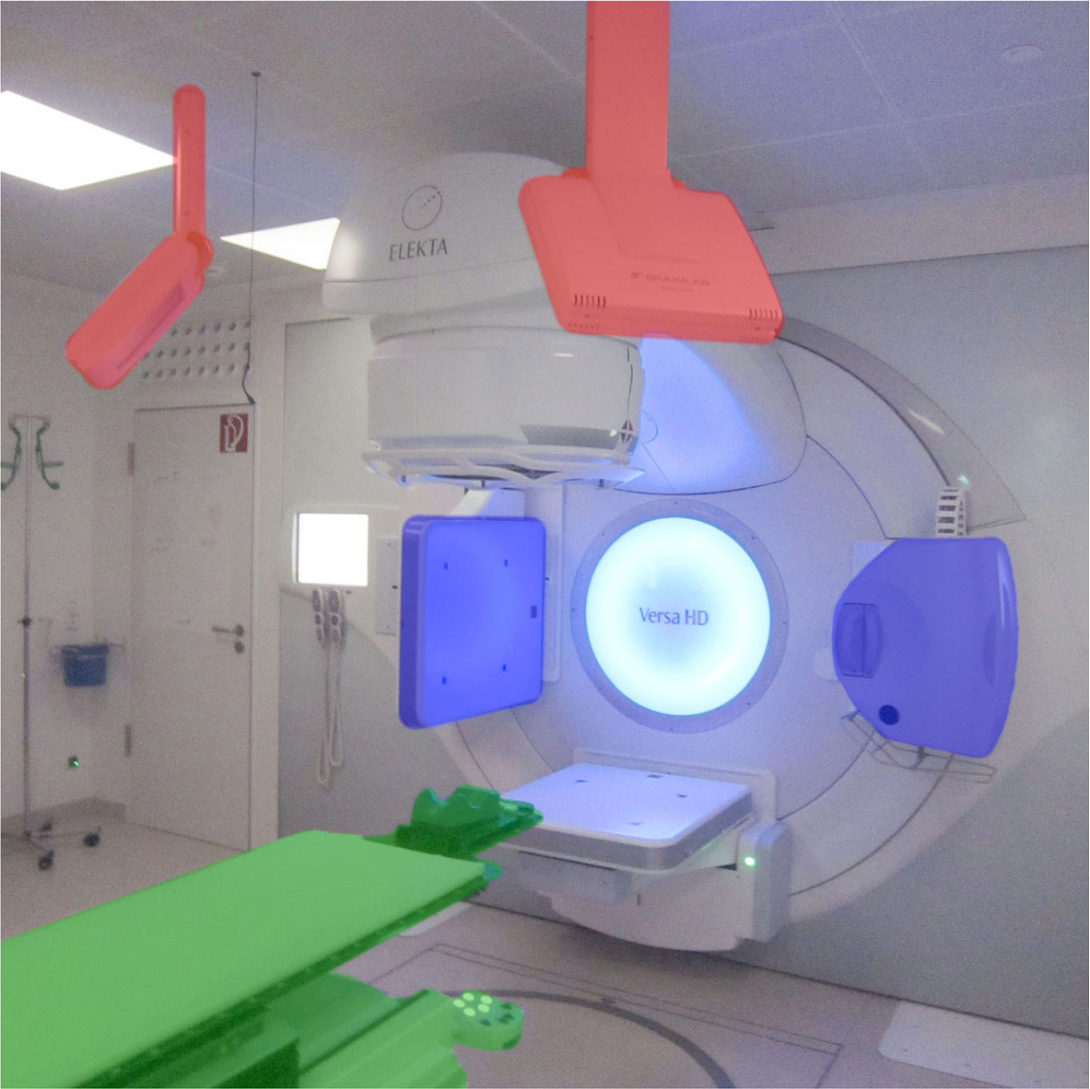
Installation of Elekta Versa HD with integrated kV-CBCT (*highlighted in blue*), Brainlab ExacTrac stereoscopic X-ray imaging (detectors highlighted in red, sources are hidden in floor) and robotic patient couch with 6 DOF Hexapod (*highlighted in green*)
